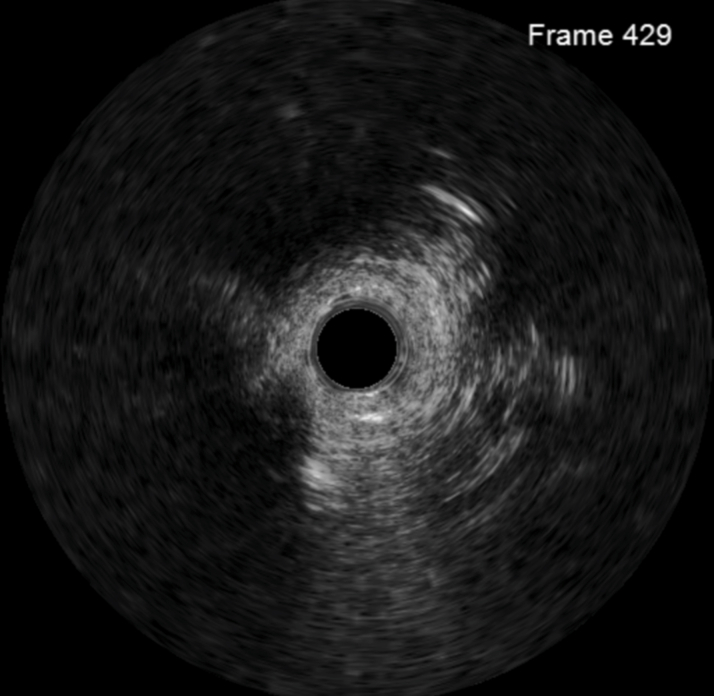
Still-Frame From Intravascular Ultrasound Following 2.0-mm Balloon Dilation Within Periaortic Adhesions Showing Complete Lack of Vascular Structure
